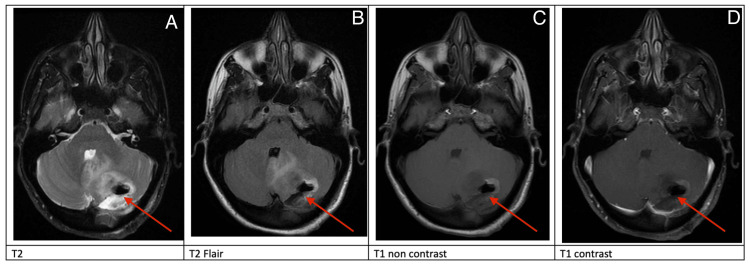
Postoperative day 1 MRI Postoperative day 1 MRI, red arrows (A-D): demonstrating gross total resection and expected postoperative changes
